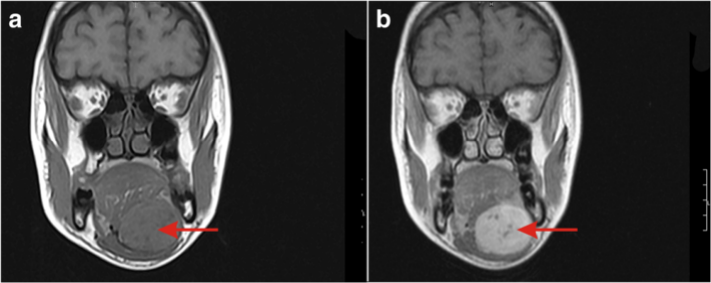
Magnetic resonance images of the patient. The image shows a well-defined contrast-enhanced round to oval mass (arrows) in the left sublingual region appearing isointense on T1-weighted images (a) and hyperintense on T2-weighted images (b).
